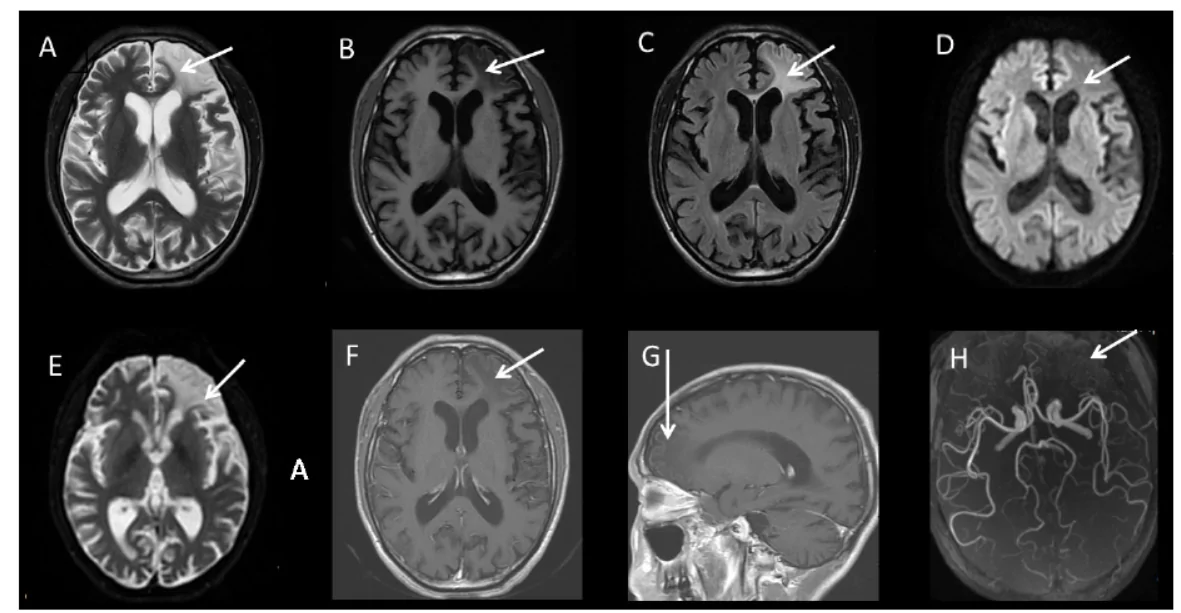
Representative images from cranial magnetic resonance imaging (MRI) of the patient. (A) T2-weighted imaging (T2WI); (B) T1-weighted imaging (T1WI); (C) T2 fluid-attenuated inversion recovery (T2-FLAIR); (D) diffusion-weighted imaging (DWI, 1000b); and (E) apparent diffusion coefficient (ADC) revealed pronounced atrophy and patchy hyperintensity zones in the left frontal lobe (white arrows), which showed no enhancement on contrast MRI (F-H) (white arrows). Magnetic resonance angiography (H) detected normal cerebral vessels.
